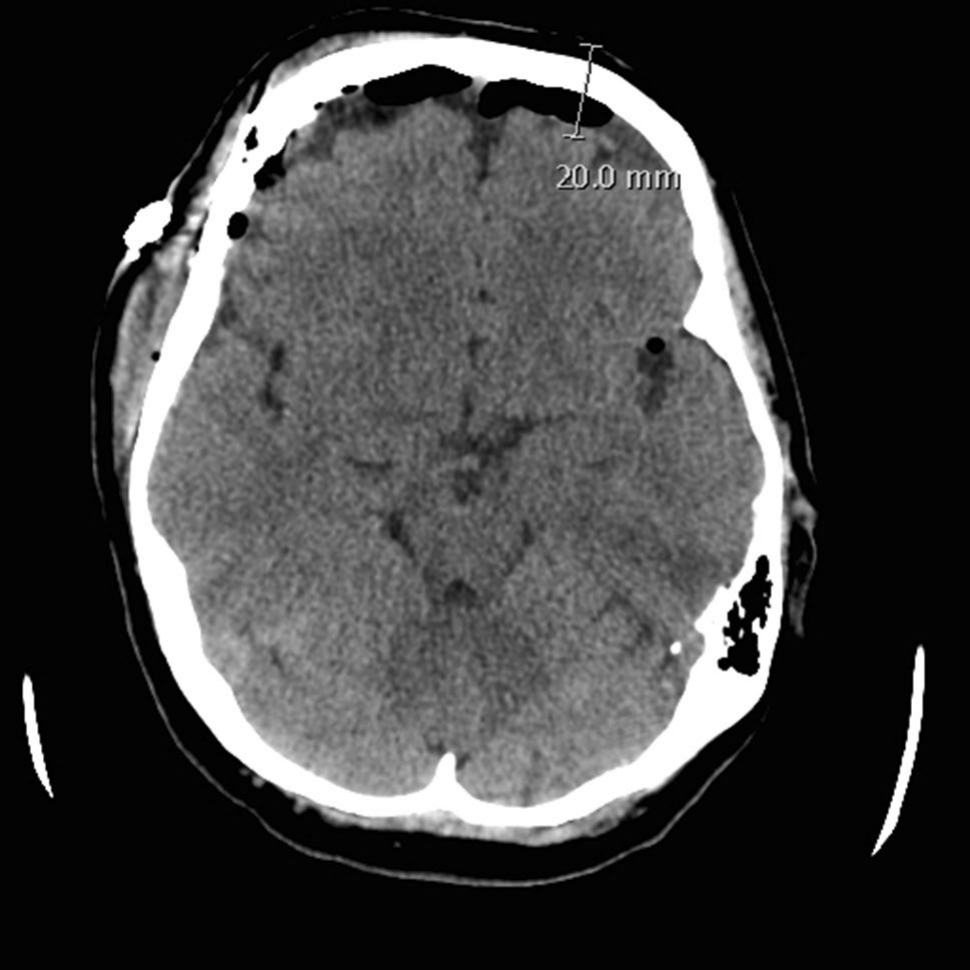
A representative postoperative axial CT scan. Distance from the skin to the cortical surface measured from the ocular papillary line 2 cm from the eyebrow
